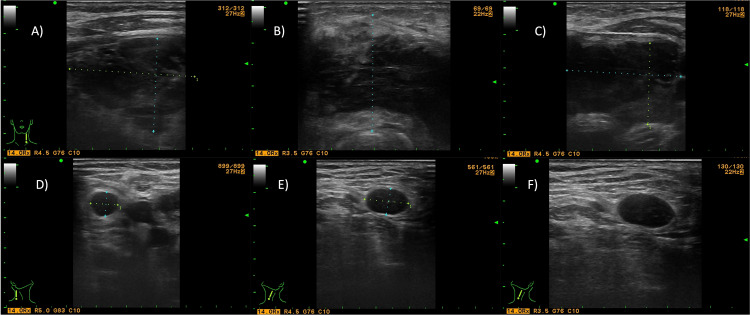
Ultrasonography at presentation Ultrasound images of the tumor formation, involving the right lobe (A), isthmus (B), and the left lobe (C) of the thyroid gland; images D, E, and F show pathological cervical lymph nodes.
